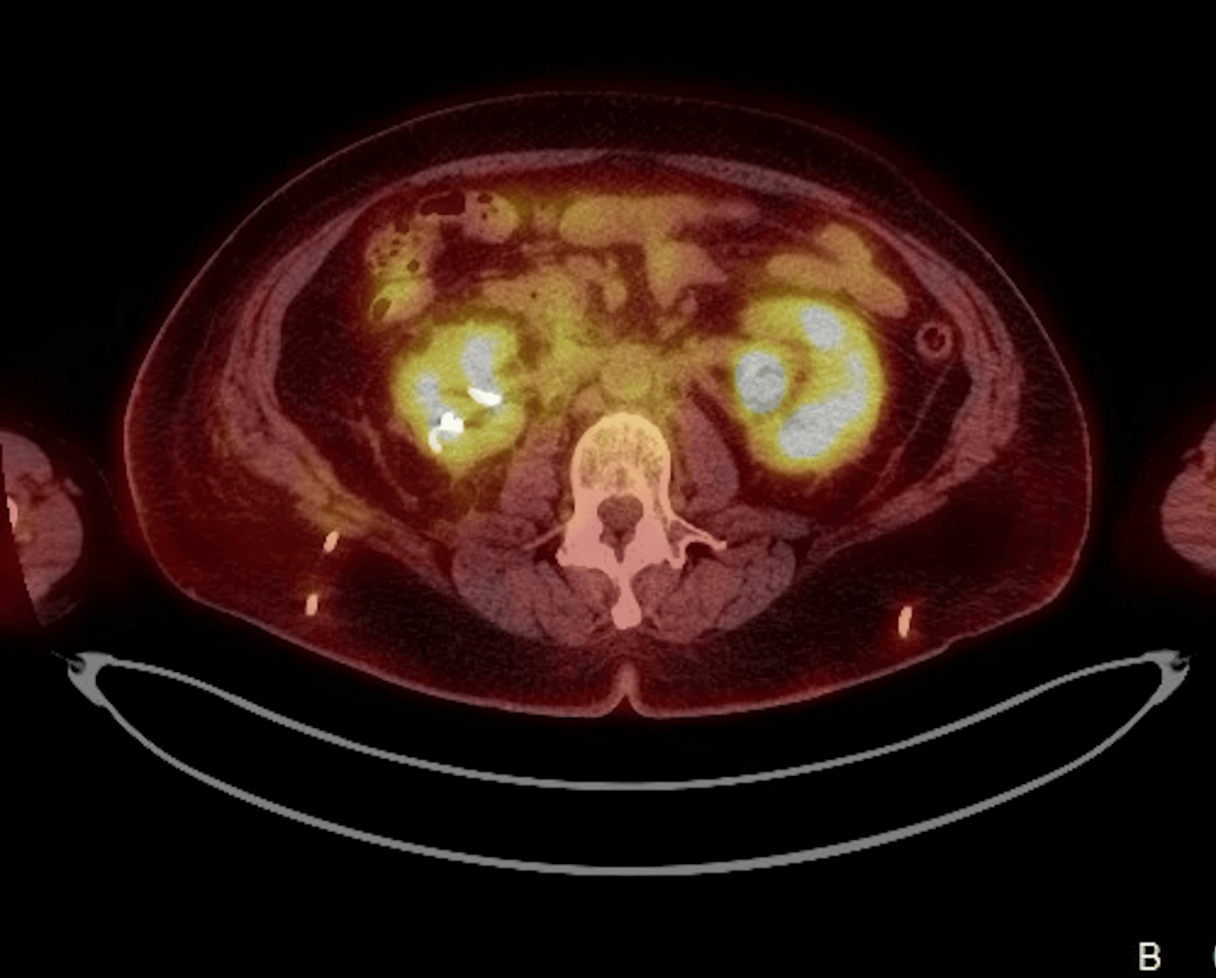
PET-CT (axial view) showing an ill-defined retroperitoneal soft tissue with mild increase in FDG activity PET-CT: positron emission tomography-computed tomography; FDG: fluorodeoxyglucose
